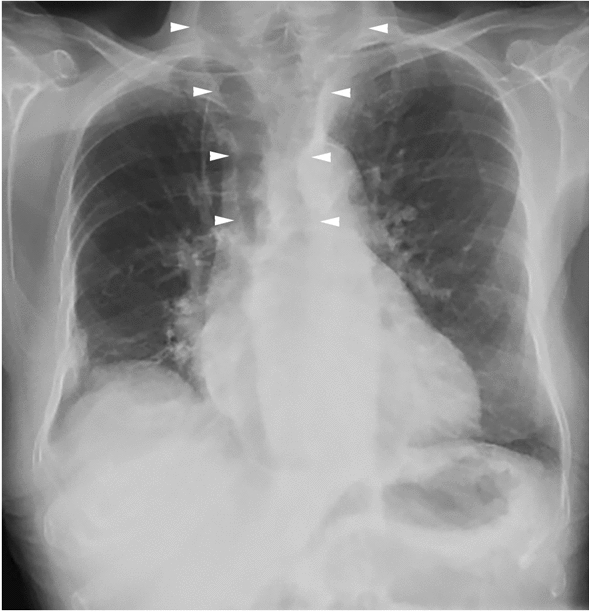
Chest X-ray in anterior–posterior direction, where the air-figure of the diverticulum is demarcated with arrowheads
